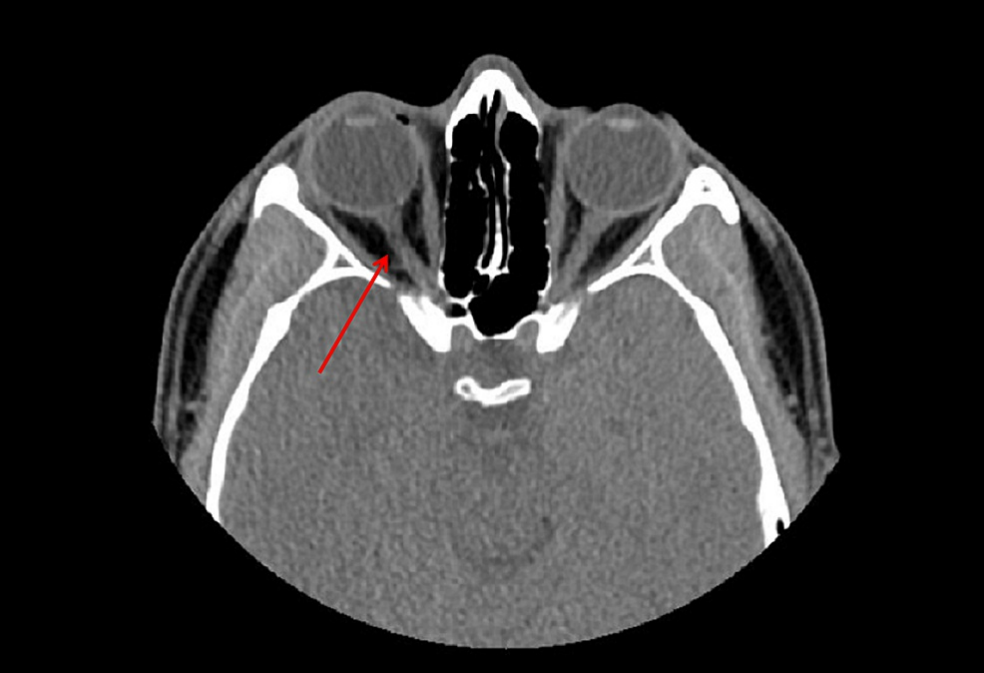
Non-contrast CT of the orbits demonstrating possible incomplete transection or compression injury of the right intraorbital optic nerve in the absence of orbital wall fractures, globe damage, or luxation.
